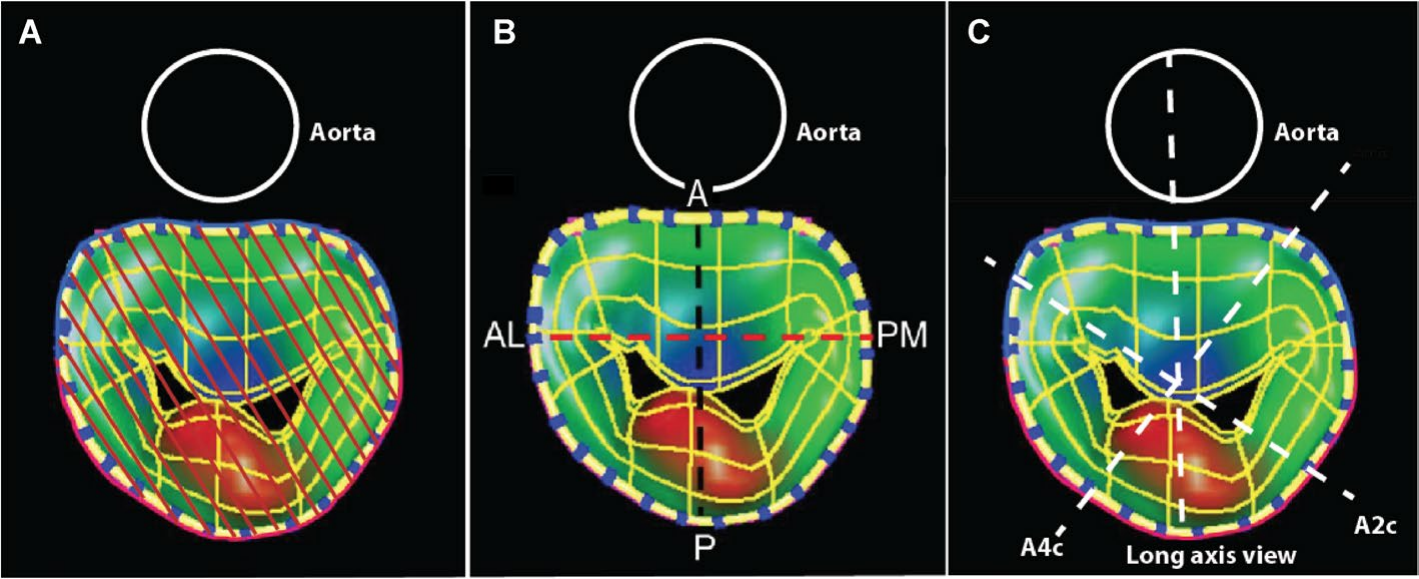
Quantitative parameters of MA. 3-dimensional shape of the MA automatically generated from a geometric model (A, B). MA area (3D TEE_sa_ MA area) (A), and anatomically correct 3D TEE AP and ALPM diameters (B) were derived. Approximate position of cut planes obtained with standard transthoracic echocardiographic views (C). A4c: apical 4 chamber, ALPM: anterolateral to posteromedian, AP: anteroposterior, A2c: apical 2 chamber, MA: mitral annulus, 3D TEE: 3-dimensional transesophageal echocardiography, 3D TEE_sa_: 3-dimensional transesophageal echocardiography semi-automated method, long-axis view: either parasternal long axis view or apical 3 chamber view
